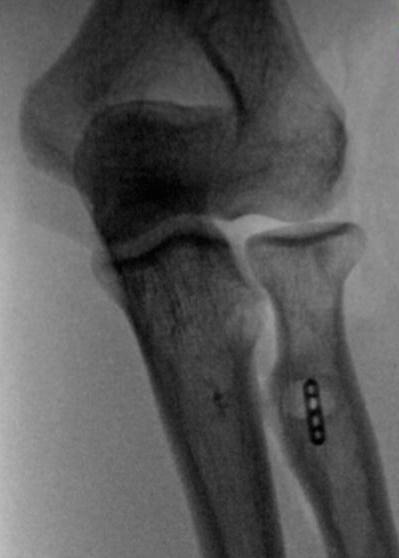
X-ray confirming correct position of cortical button at level of bicipital tuberosity.
